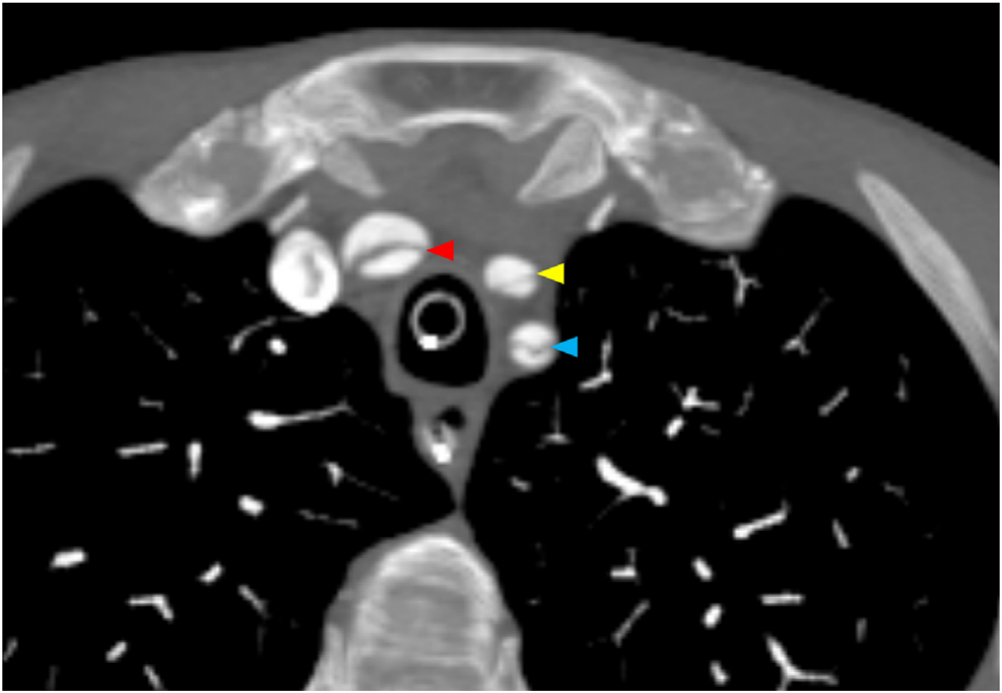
Transverse computed tomography angiography of the neck showing dissection flaps (arrowheads) in the brachiocephalic (red), left common carotid (yellow), and left subclavian (blue) arteries.
